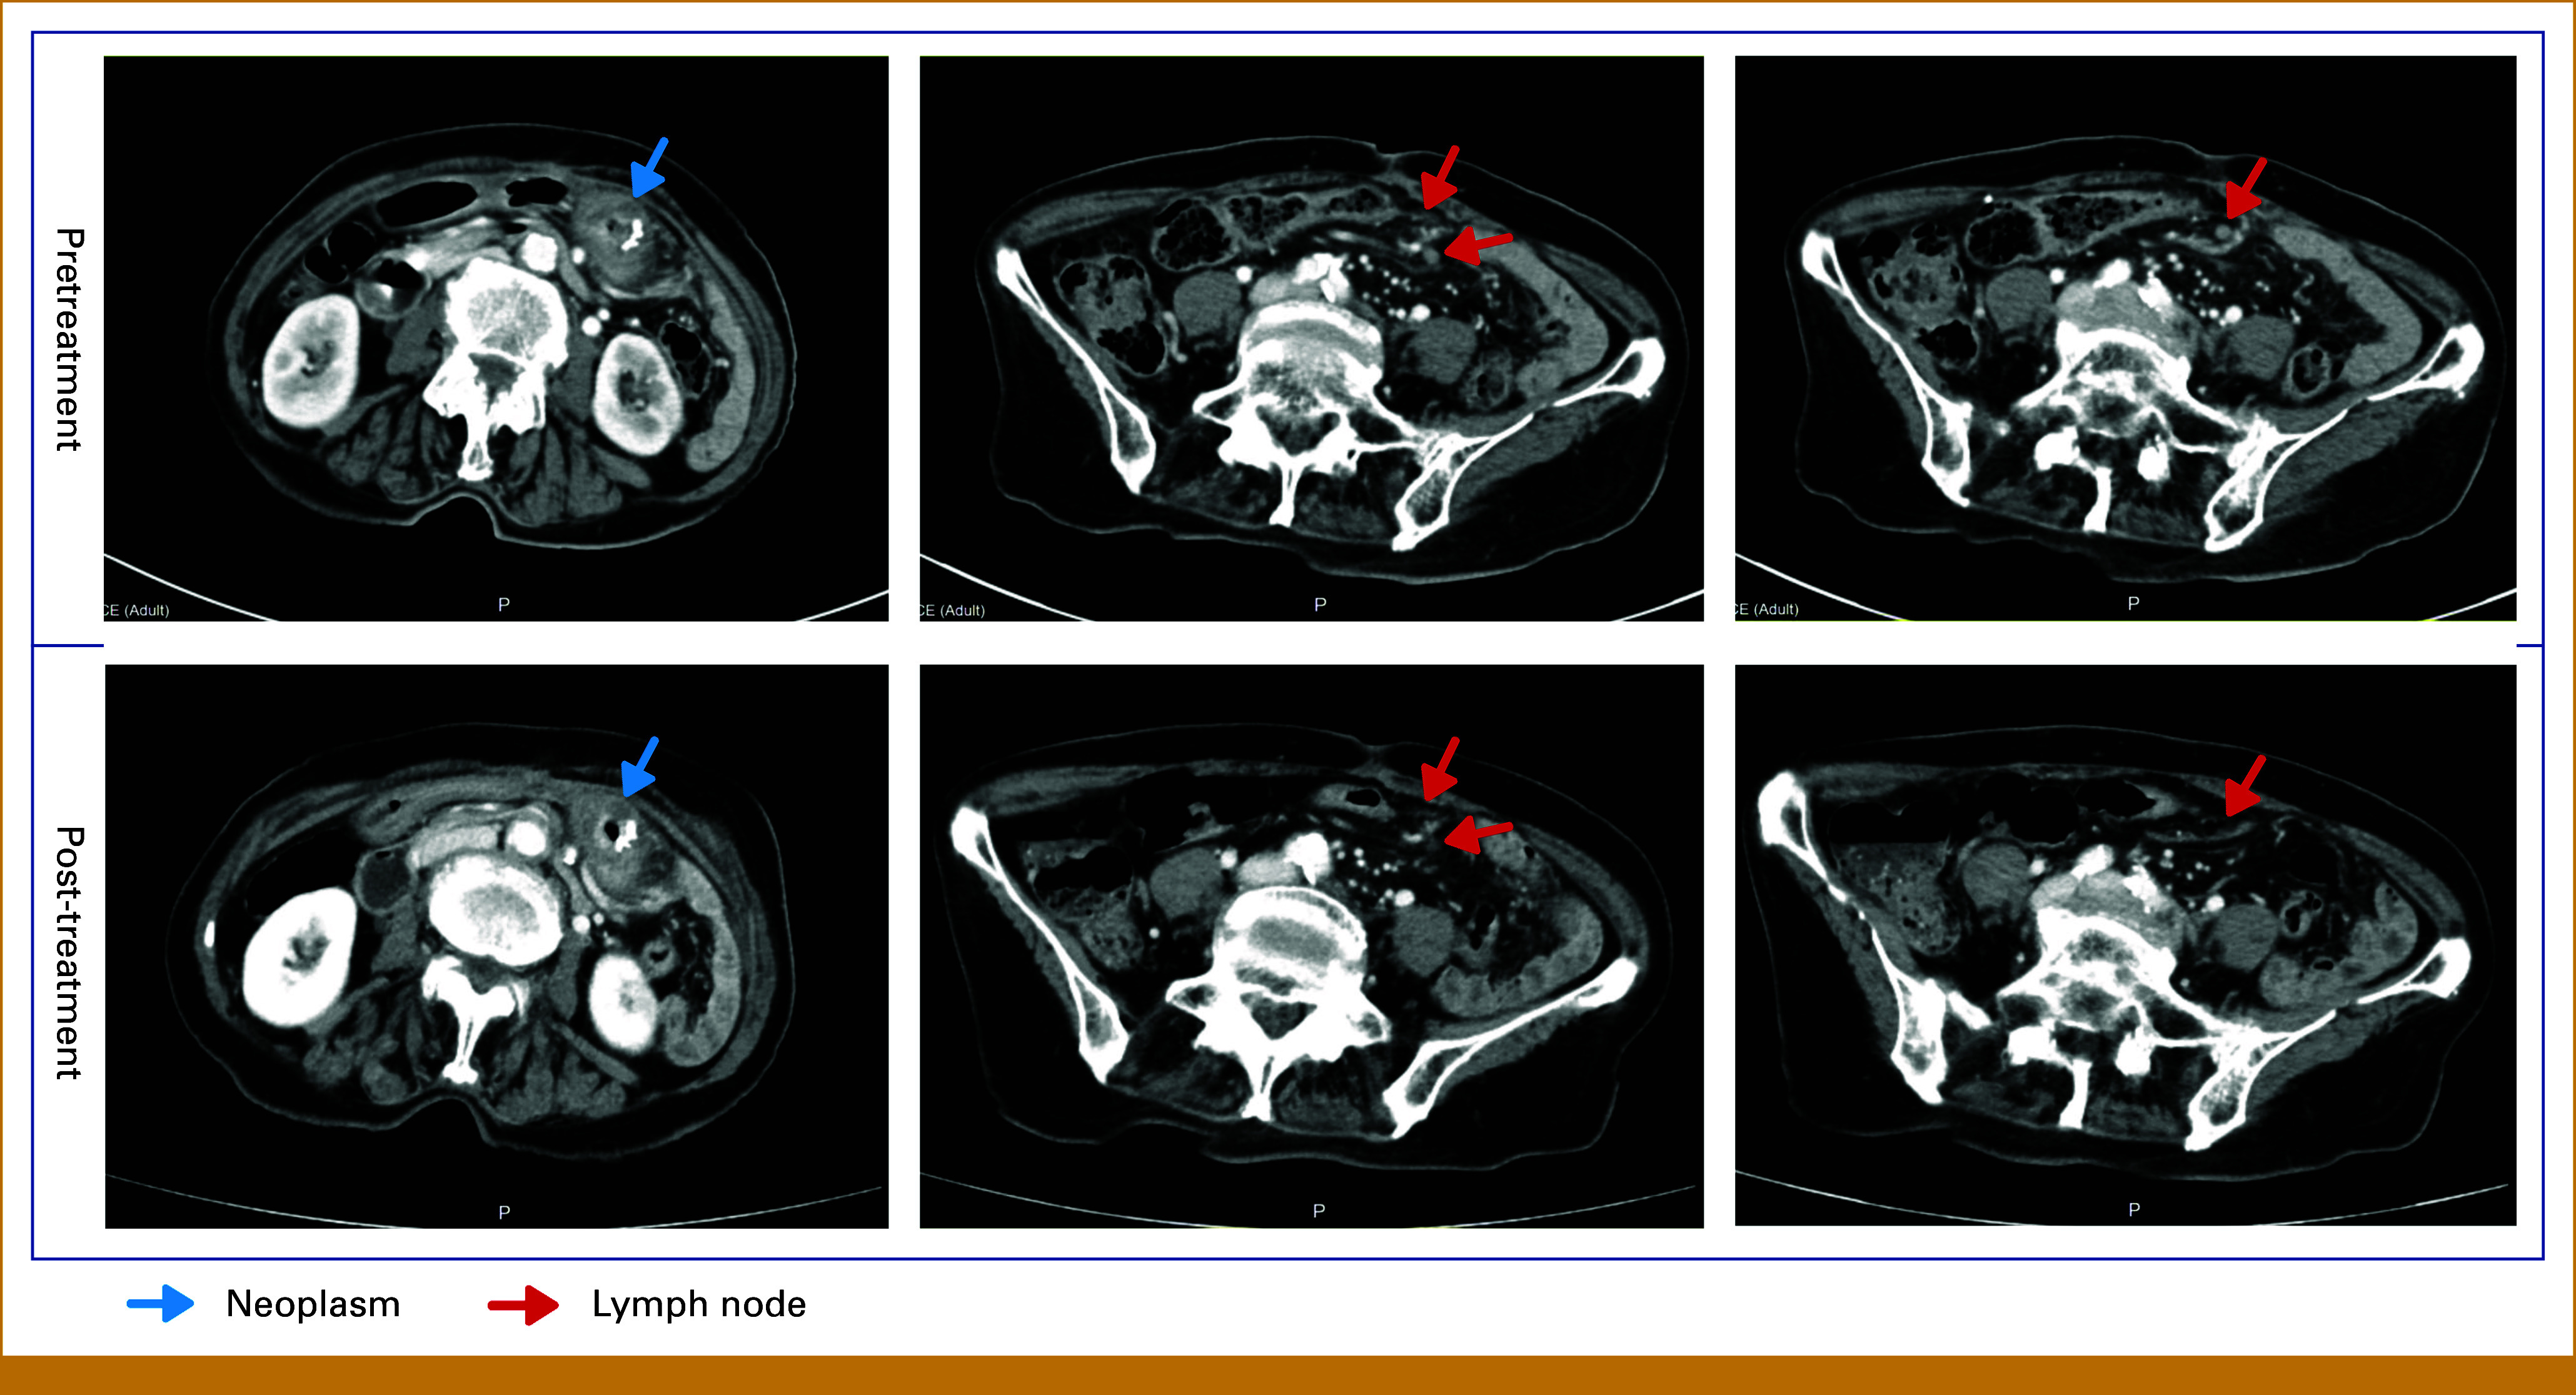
Computed tomography before and after 2 weeks of treatment with selpercatinib.
